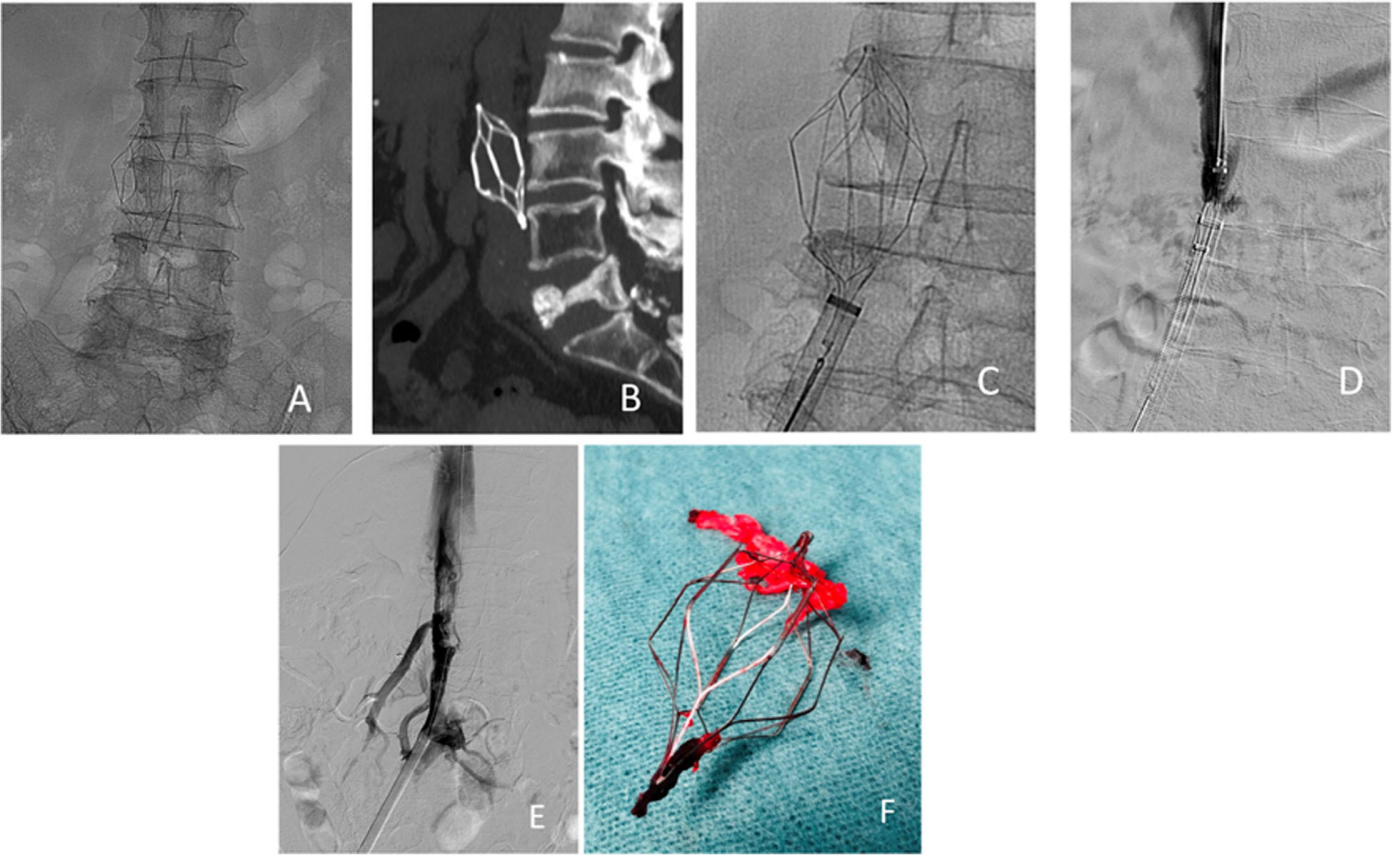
Example of IVCF retrieval with difficulty. A 61-year-old female patient diagnosed with multiple myeloma. Six years ago she had DVT in her left lower limb. She was treated with unfractionated heparin and had an upper-GI bleed, and anticoagulants became contraindicated. An Optease filter was implanted infrarenal. a. abdominal x-ray showed the Optease filter in a proper position. b. Abdominal CT with sagittal MPR showed the filter slightly tilted on the vertical axis. c. Femoral access with a 16 Fr sheath and a 25 mm snare (Amplatz GooseNet snare). After several attempts, it was impossible to recover it. d. Jugular access was made with a 12 F sheath and a guide was passed through the upper vertex of the filter and with several movements from both accesses, the IVCF was introduced into the sheath and the filter could be recovered. e. Cavography after filter retrieval (shows endothelial alteration). f. The filter recovered with endothelial remains
